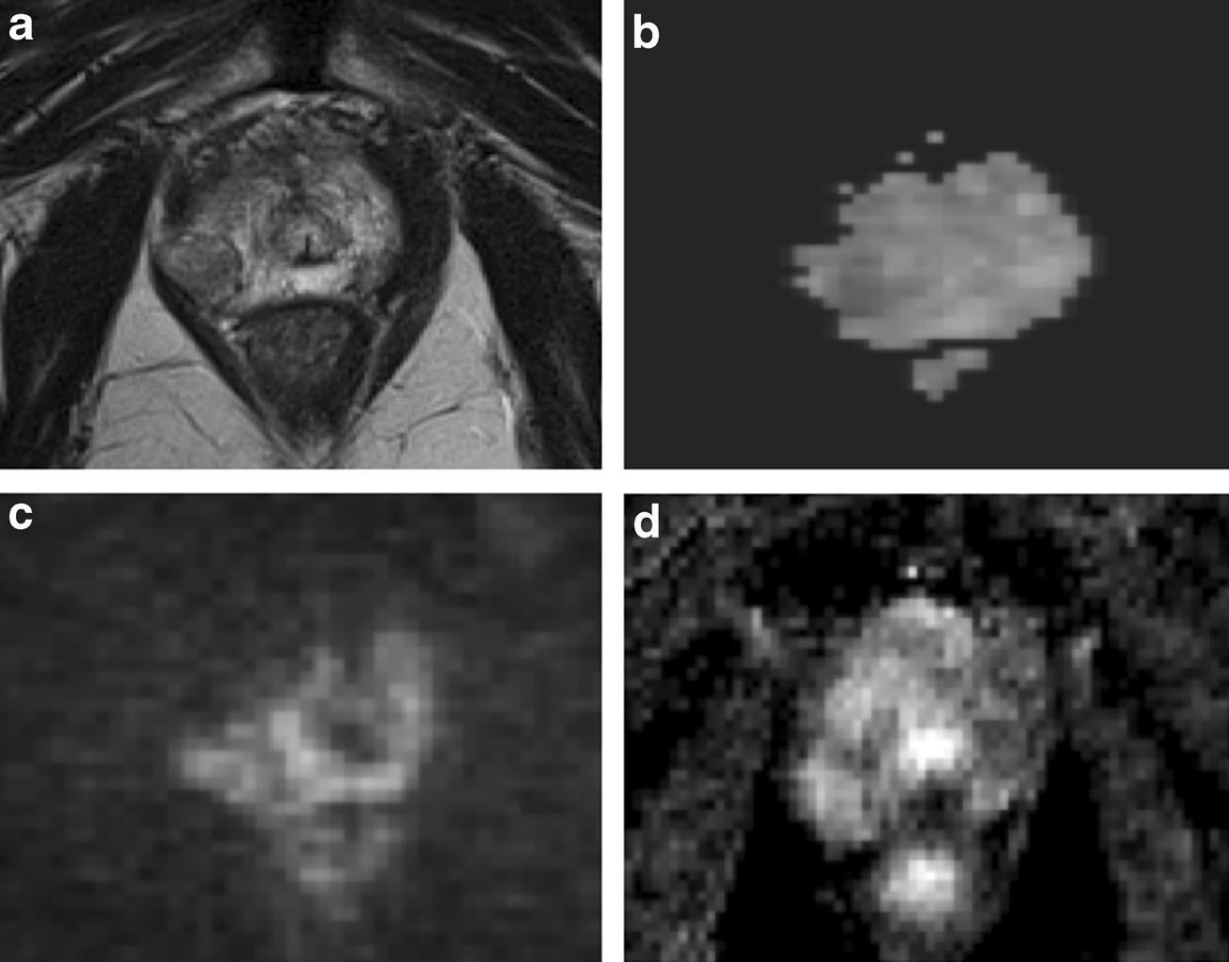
Ectopic nodule in the right peripheral zone with no ERC. a Axial T2-weighted fast spin-echo image showing an hypointense well circumscribed nodule in right peripheral zone with an evident peripheral capsule. b ADC map and c axial DWI image with b value = 3000 mm^2^/sec, showing no significant restriction of water diffusivity. d Axial perfusion gradient-echo T1-weighted image shows marked and early enhancement simultaneous to other nodule constituents of the adenoma
